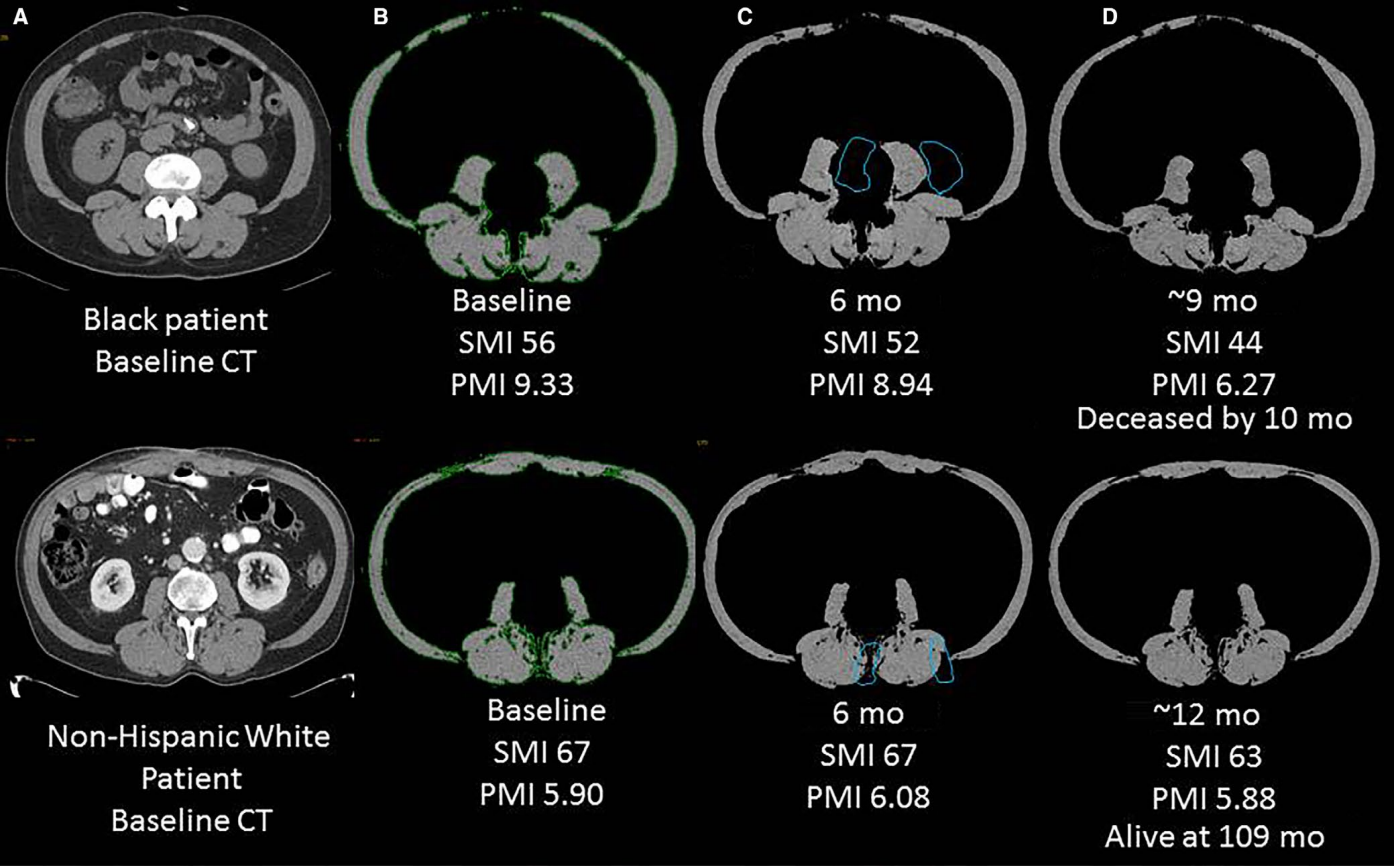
Axial CT images for a Black (top panel) and NHW PC patient (bottom panel). A, Baseline/preoperative image at the inferior endplate of L3 vertebral body. B, Axial image after skeletal muscle is segmented (in green) and non‐muscular structures are excluded using a HU threshold of −29‐+150. C and D, six and 12 mo postoperative follow‐up CT exams showed downward trends in SMI and PMI, especially for the Black patient. Psoas muscle area is outlined in blue on images. Skeletal muscle and psoas muscle areas were calculated on each exam and indexed for patient's height. Note: The NHW patient has higher baseline SMI but lower PMI than the Black patient which can be explained by the relative smaller size of psoas muscles with respect to other skeletal muscles. CT, computed tomography; HU, hounsfield unit; NHW, Non‐Hispanic White; PC, pancreatic cancer; PMI, psoas muscle index; SMI, skeletal muscle index
